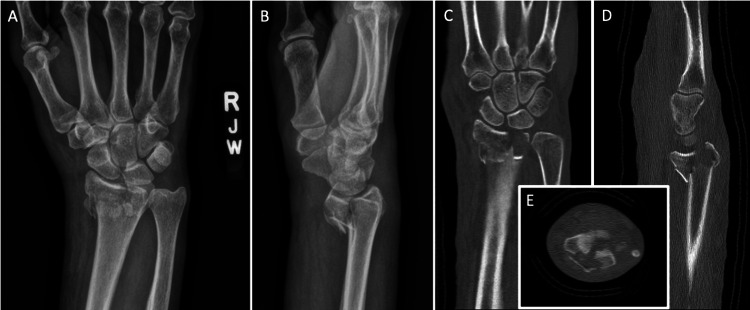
Injury films and preoperative CT scan A-B) anteroposterior and lateral X-rays views of the right wrist: comminuted volar Barton distal radius fracture with loss of radial height and volar radial tilt. C-E) coronal, sagittal, and axial views of CT scan showing comminuted fracture pattern, depressed articular surface.
